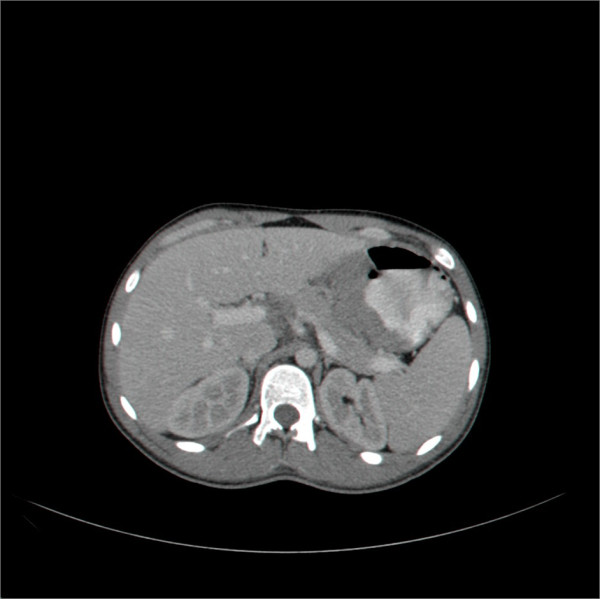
Findings in abdominal computed tomography consisting of a gastric mass.
